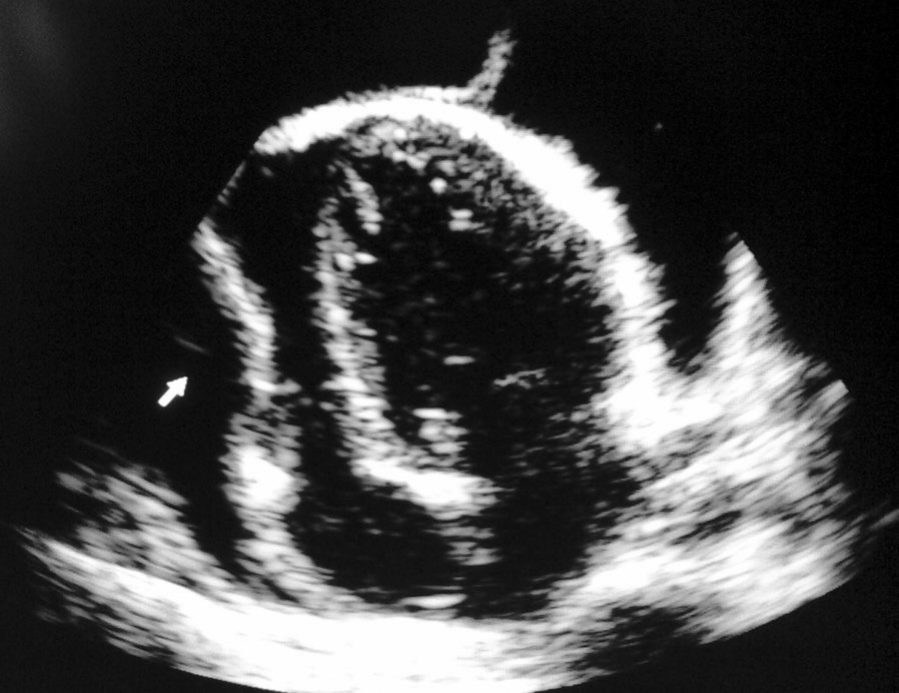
Echocardiogram of a subject with pericardial effusion
